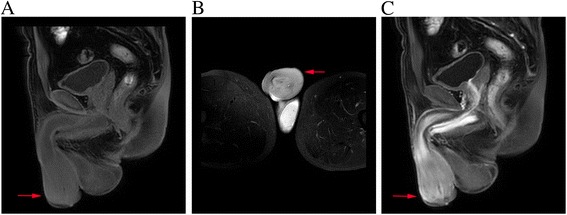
a Sagittal T1-weighted magnetic resonance imaging scan showing mass around the corpus cavernosum (arrow); b Axial T2-weight magnetic resonance imaging scan showing ill-defined mass around the corpus cavernosum (arrow); c Sagittal T1-weighted fat suppression contrast-enhanced magnetic resonance imaging scan showing enhanced mass around the corpus cavernosum (arrow)
